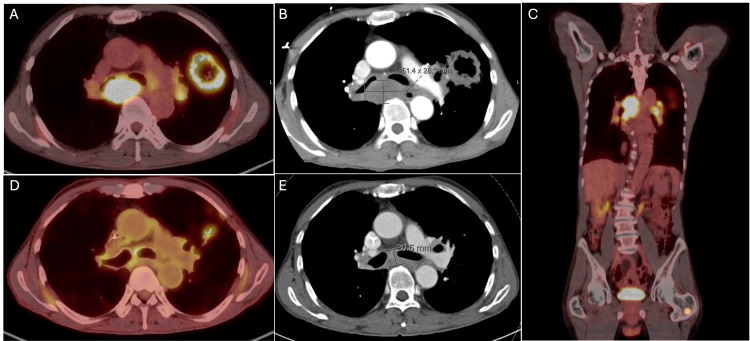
Oligo-metastatic adenocarcinoma of the lung The pre-chemotherapy imaging shows the fluorodeoxyglucose (FDG) avid mass in the left lung and mediastinum seen on (A) axial PET-CT, (B) axial CT, and (C) sagittal PET-CT, also showing a left femur metastasis. Subsequent post-chemotherapy imaging revealed a decrease in the size and FDG avidity of mediastinal and left lung disease on (D) axial PET-CT and (E) axial CT. PET-CT: positron emission tomography/computed tomography.
